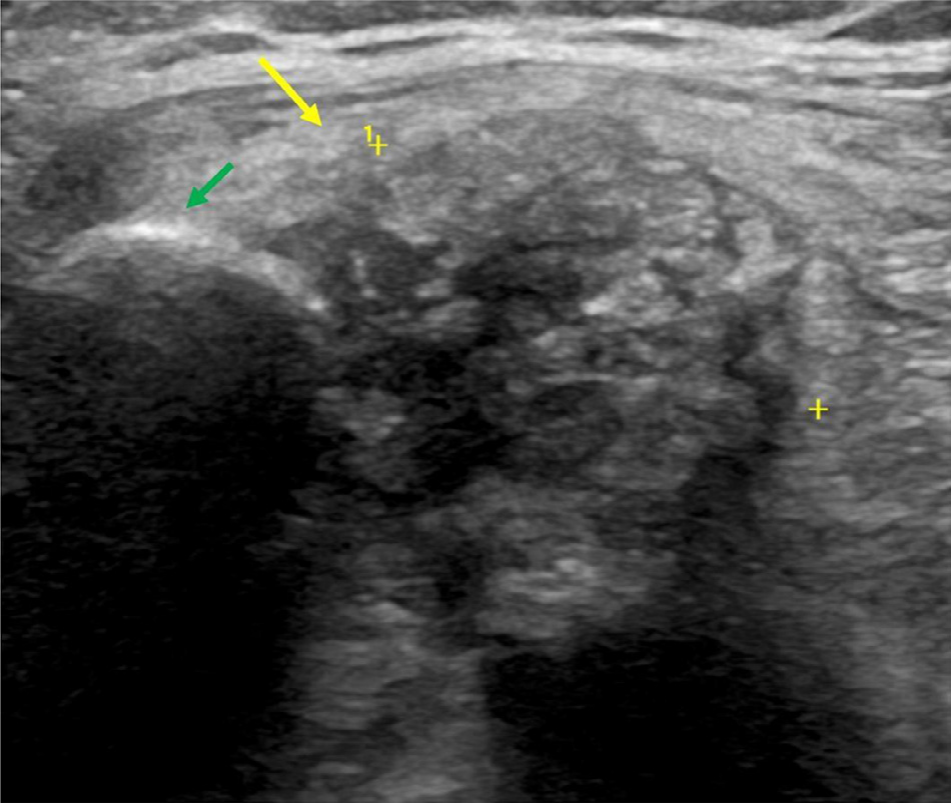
B-Mode ultrasound image in the transverse plane which confirms the presence of an ovoid mass containing hyperechoic images within, suggestive of calcifications (yellow arrow) in close contact with the clavicular bone (green arrow).
